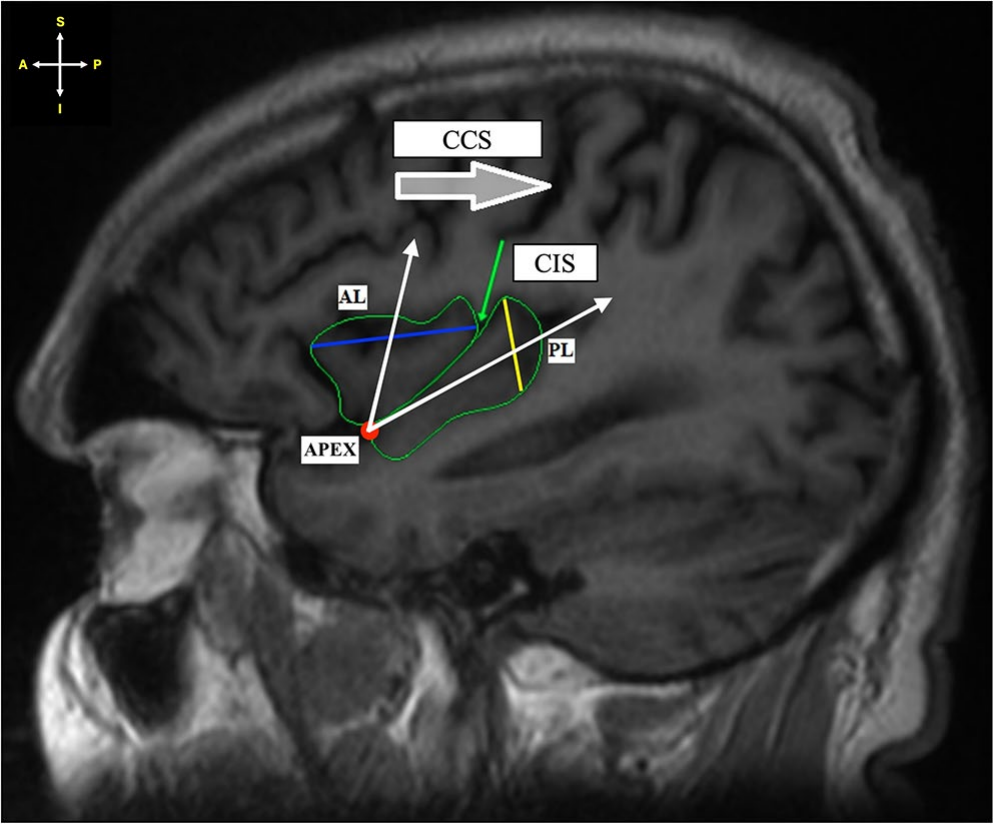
Sagittal section of MRI showing the maximum width of each lobule. *Key* AL - Anterior lobule; PL - Posterior lobule; CCS - Central cerebral sulcus; CIS - Central insular sulcus. The white lines represent the directional axis of each lobule
